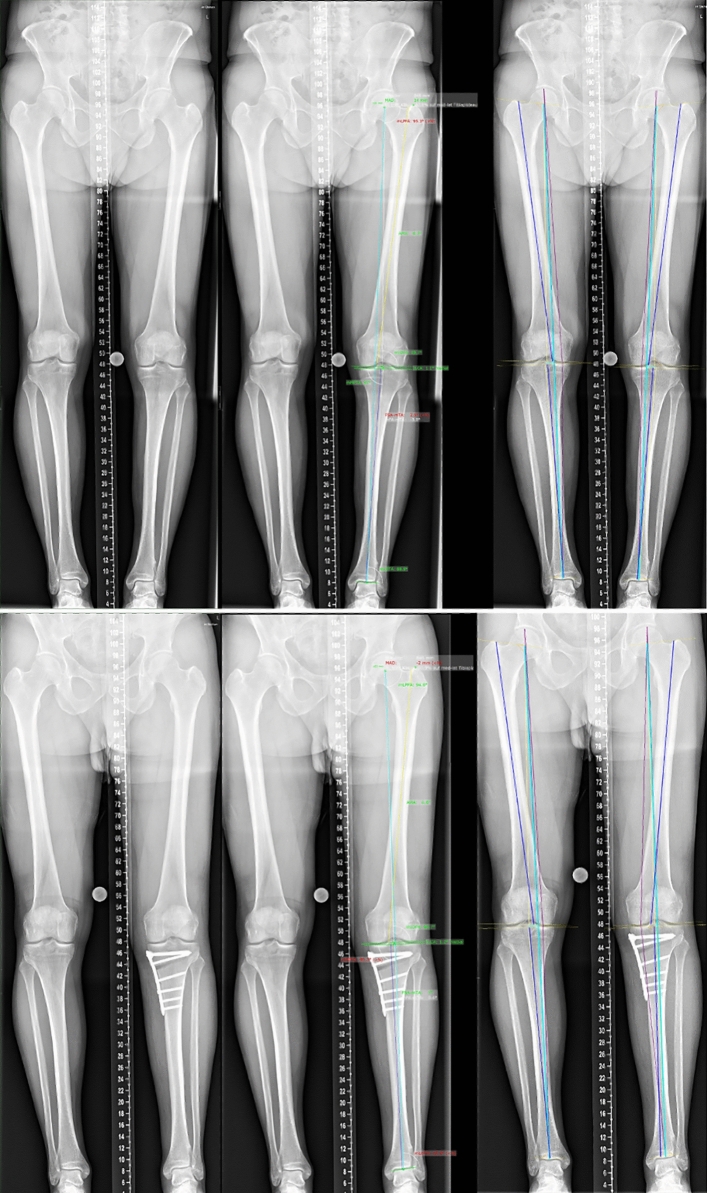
Representative images of weight-bearing long-leg radiographs of a patient with mild varus malalignment (top) and after medial opening wedge osteotomy (bottom). The figure shows native X-rays (left), manual measurements using mediCAD® (center), and fully automated analysis performed by an AI software (LAMA™); right). Measurements include mechanical axis deviation (MAD), hip–knee–ankle angle (HKA), joint line convergence angle (JLCA), mechanical lateral distal femoral angle (mLDFA), and medial proximal tibial angle (MPTA)
